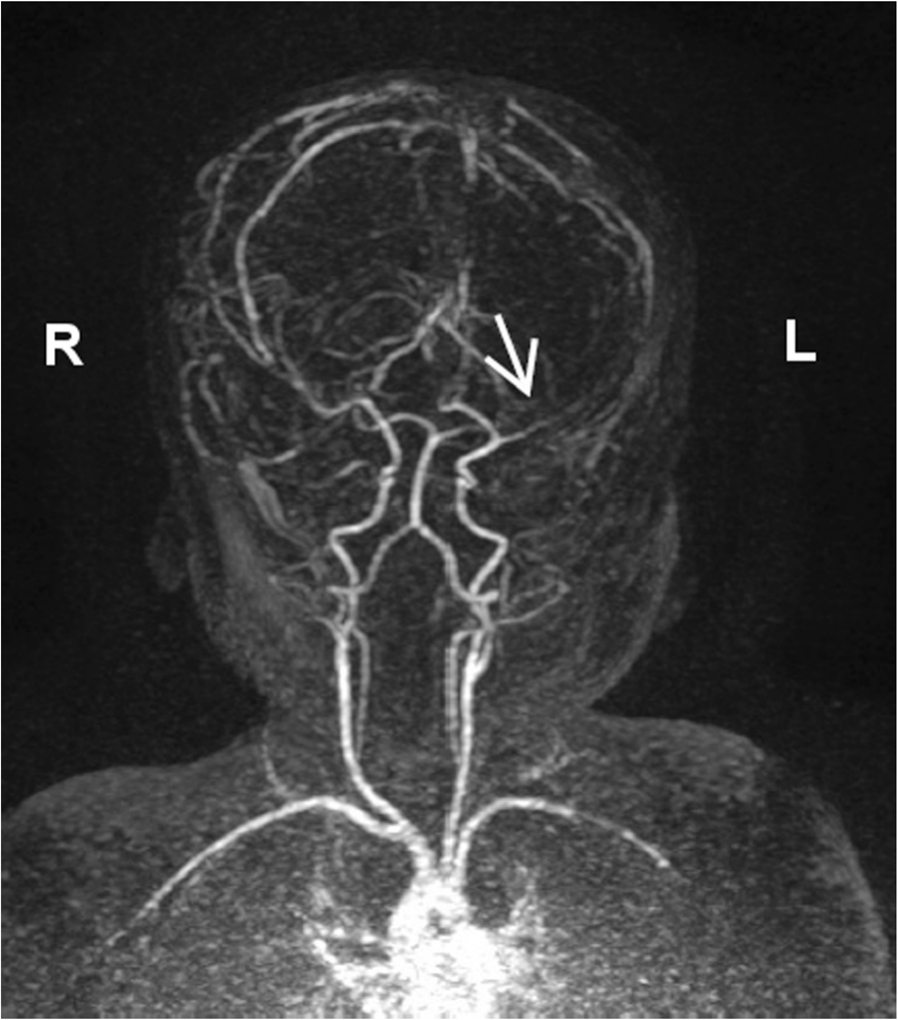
Two-month-old patient. Contrast enhanced-MRI-angiography. Three-dimensional multiplanar reconstruction image shows normal patency of the carotid arteries and hypoplasia of the left middle cerebral artery (white arrow).
